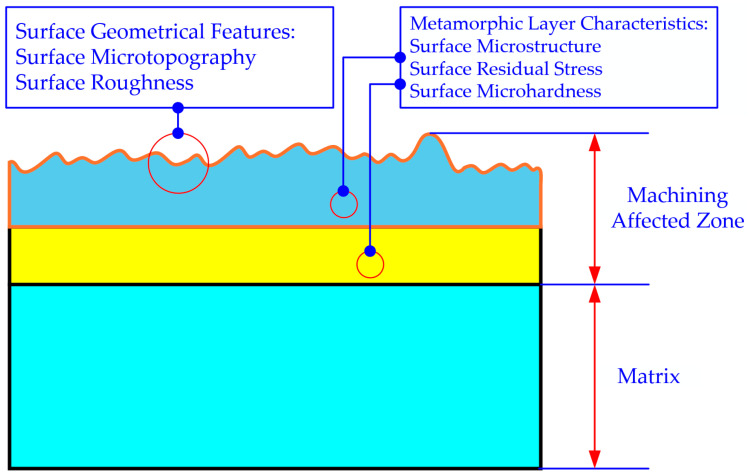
Study indicators for surface integrity of GH4169 in ultrasonic elliptical vibration cutting.
